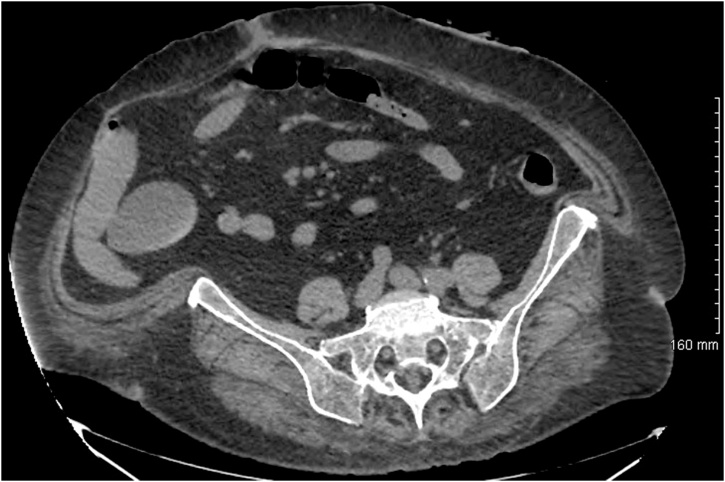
Preoperative abdominal CT with enormous recurrence of incisional hernia along the right iliac fossa.
